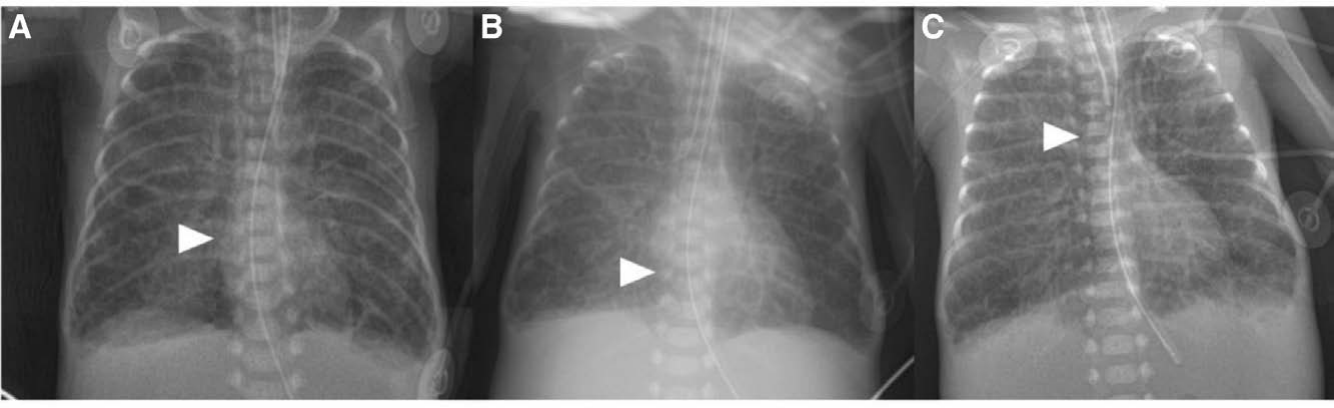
Chest radiographies which were taken before and after cardiopulmonary instability, case 2 patient. Chest radiography was taken on the morning, before the cardiopulmonary deterioration. The distal tip of PICC line lied in border of cardiac silhouette (A), chest radiography immediately after sudden onset of cardiopulmonary deterioration, which showed cardiomegaly with pulmonary congestion compared to the morning chest radiography. The distal tip of PICC line was located in right atrium (B), chest radiography taken after repositioning the PICC, after pericardiocentesis during cardiopulmonary resuscitation. The distal tip of PICC is placed outside of cardiac silhouette. The distal tips of PICC line were indicated by arrow. PICC = peripherally inserted central catheter.
